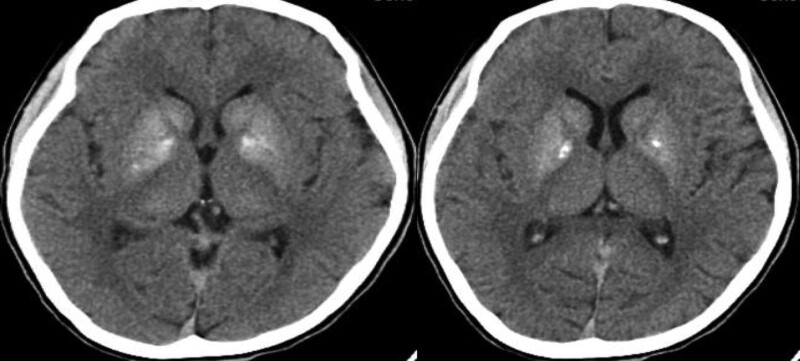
Computed tomographic scan demonstrating symmetrical high-density shadows in both basal ganglia regions.
